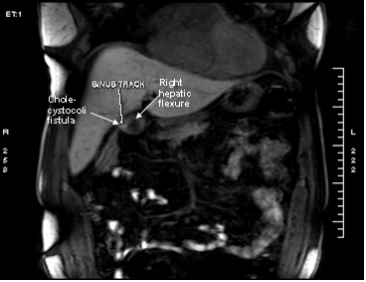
MRI:T1 and T2 weighed images with iv contrast Gadolinium- Bopta, revealing fistulous tract between the right colonic flexure and gallbladder (cholecystocolic fistula) and a large gallstone (2 cm).
